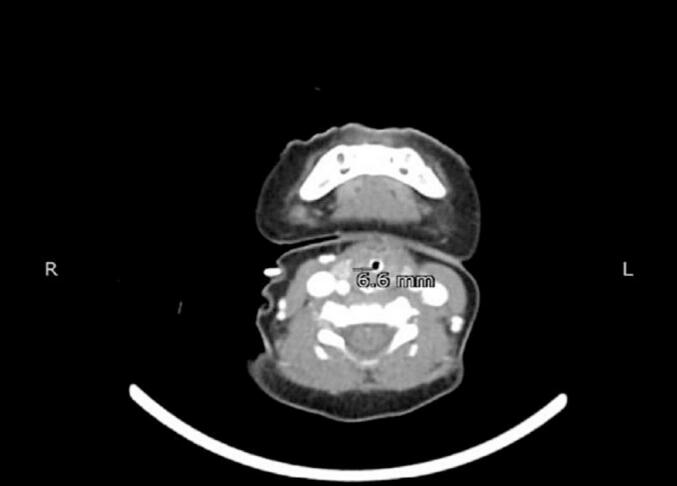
CT scan of neck (axial view).
